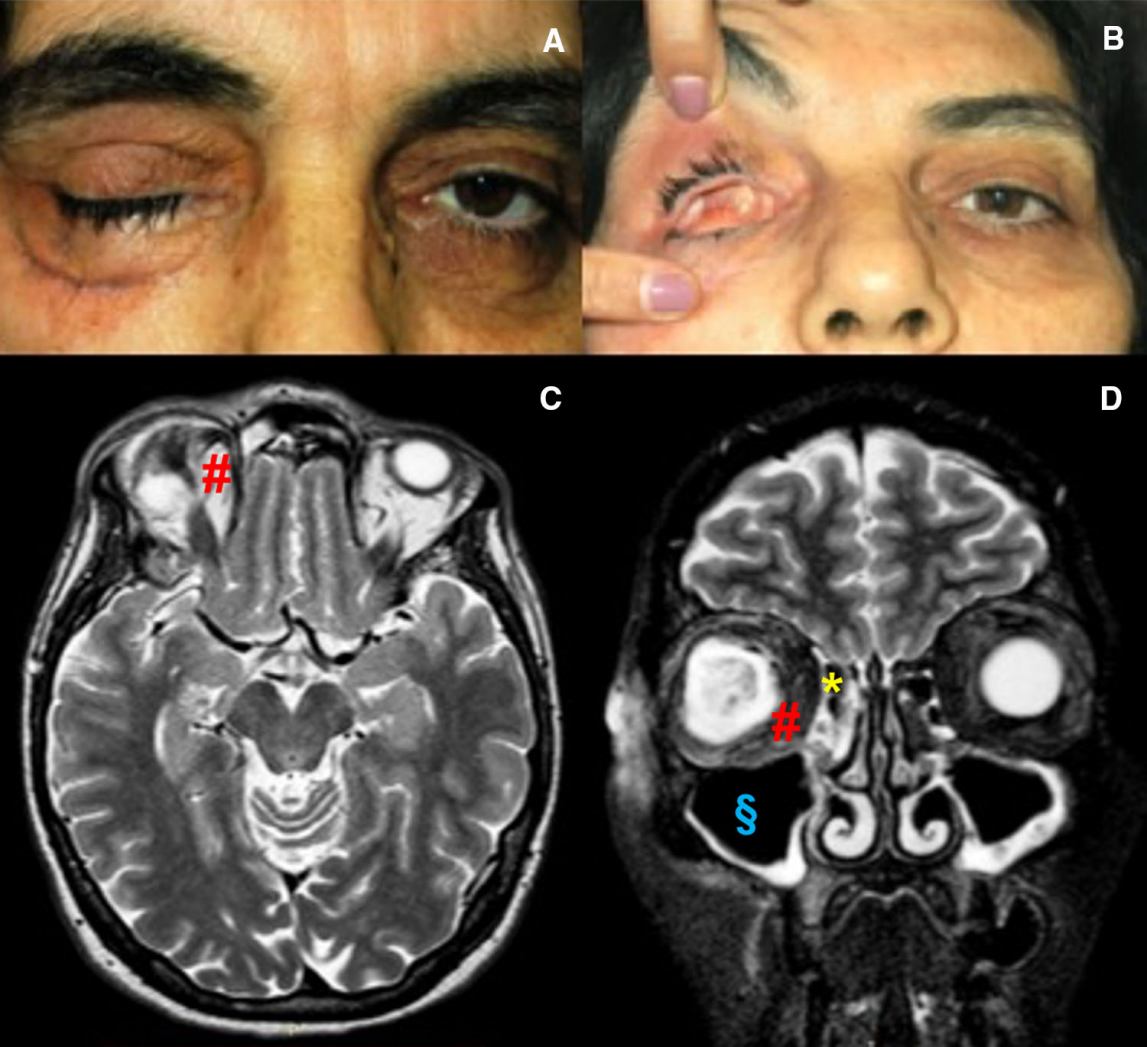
Case 3: Postsurgical photo of patient in frontal section: closed eyelid (a), open eyelid showing orbit evisceration (b), MRI in Axial section (c) and MRI Coronal section (d): ethmoid sinus drained of purulent secretions (*), maxillary sinus totally empty (§), resolution of the orbital abscess (#)
